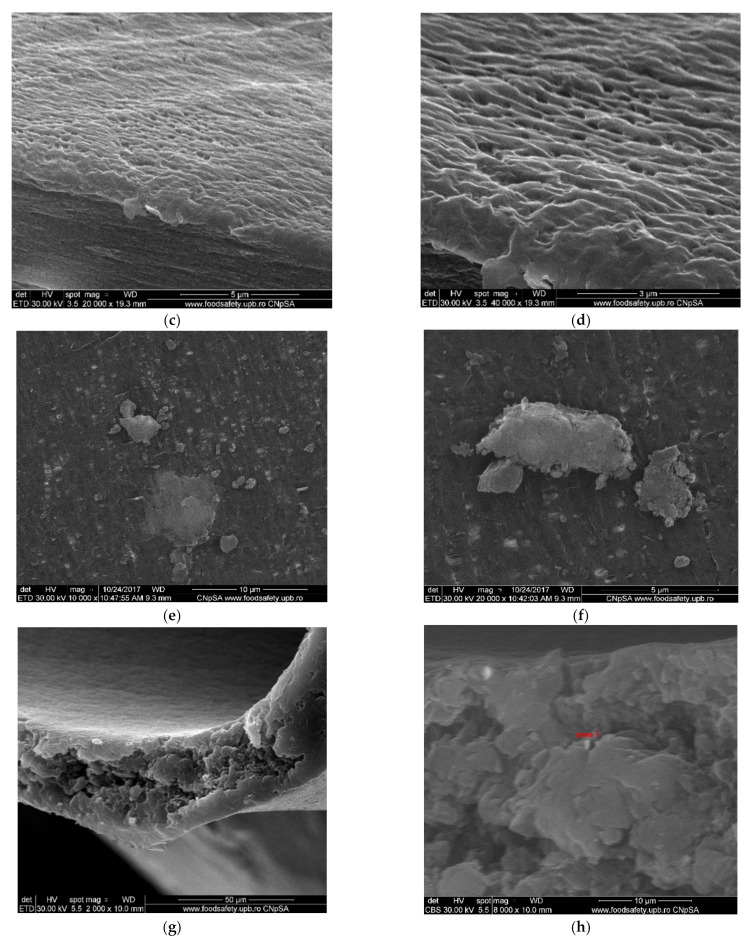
SEM morphology of the membranes: (a) membrane support (PPMS); (b) membrane support (PPMS) detail; (c) impregnated membrane (Ag-Cell-Ac-PPM); (d) impregnated membrane (Ag-Cell-Ac-PPM) detail; (e) processed impregnated membrane (Ag-Cell-Ac-PPM); (f) processed impregnated membrane (Ag-Cell-Ac-PPM) detail; (g) cross-section on Ag-Cell-Ac-PPM; (h) detail of the cross-section on Ag-Cell-Ac-PPM with the evidenced Energy Dispersive X-Ray Analysis (EDX) zone.
